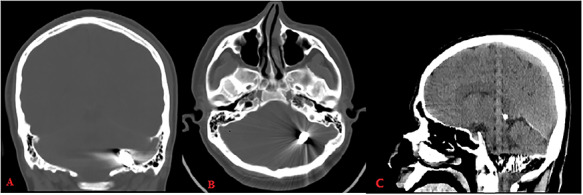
Computed tomography (bone window: A: coronal, B: axial) reveals metallic object located between left occipital bone and inferior side of left cerebellar hemisphere; CT (brain window C: sagittal) shows hemorrhage along its trajectory.
